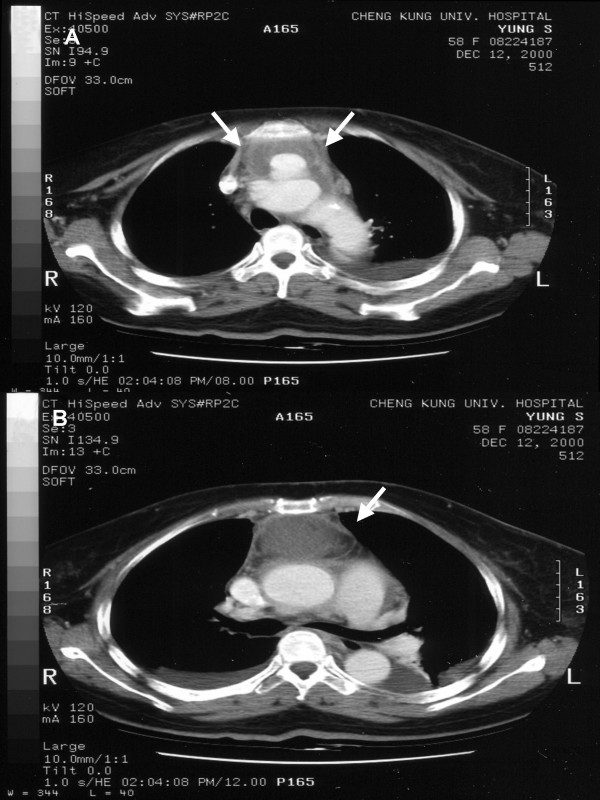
Axial CT scans. (A, B) Images of upper mediastinum show pseudoaneurysm with periaortic infiltration (white arrow).
